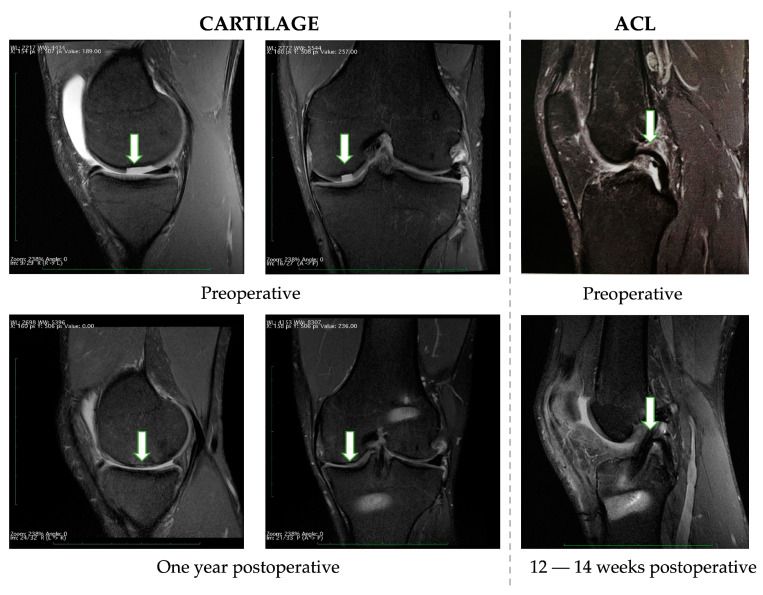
Preoperative and one-year postoperative MRI data demonstrating cartilage regeneration progression (the arrows show the cartilage defect and marrow edema before surgery, and the healed defect and withdrawn marrow edema one year later). 12–14 weeks postoperative MRI on the right side demonstrated full remodeling of the ACL graft.
